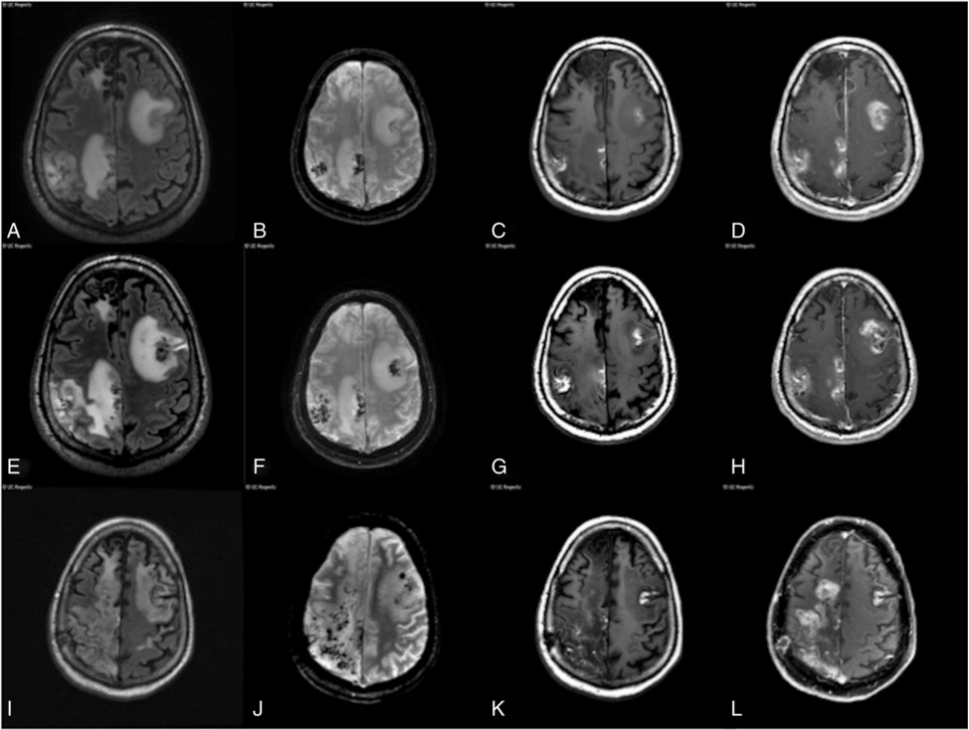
Magnetic resonance images from 2012. a-d Axial fluid-attenuated inversion recovery (FLAIR), axial 3D gradient recalled, susceptibility-weighted image, axial pre-contrast T1, and axial post-contrast T1-weighted images on June 12, 2012. e-h same sequences on June 19, 2012. i-l same sequences on August 12, 2012
